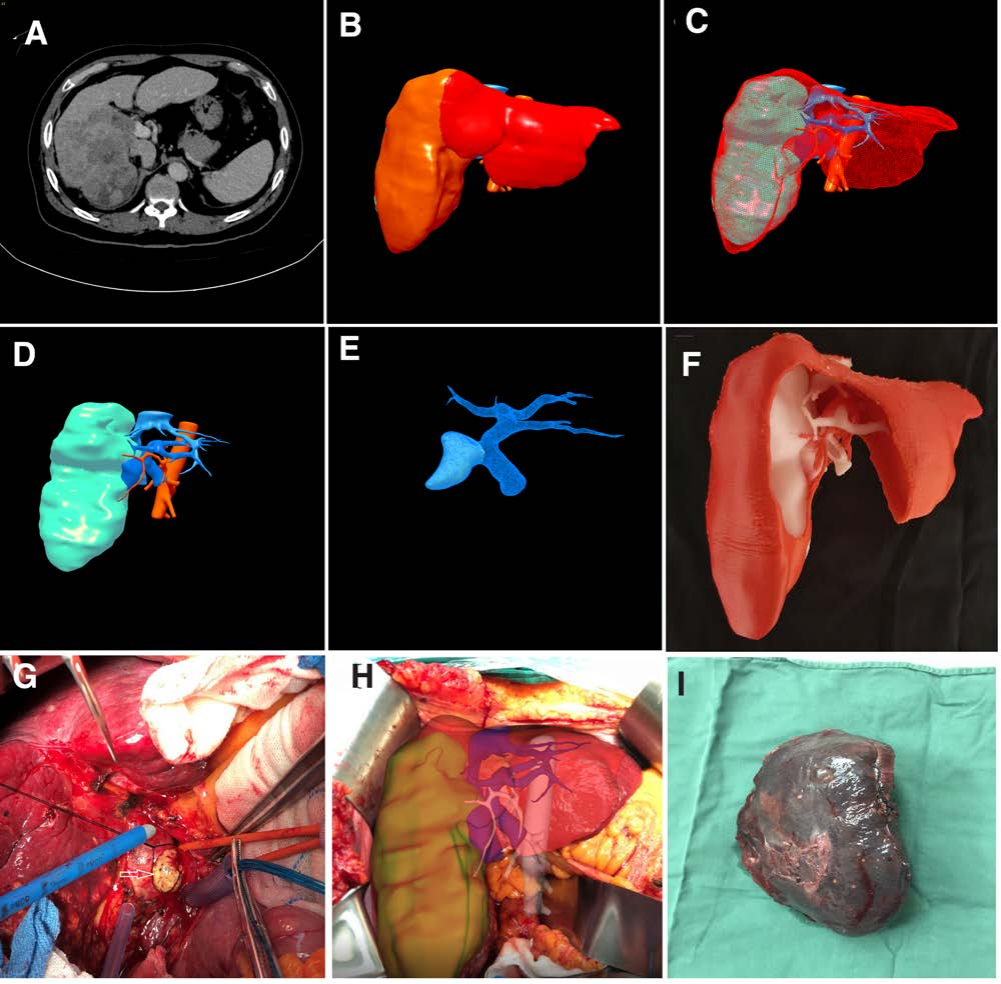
Preoperative evaluation and surgery navigation of liver cancer with portal vein tumor thrombus (PVTT). (A) The CT image showed right liver cancer with PVTT and liver fibrosis. (B) A three-dimensional (3D) reconstruction model showed the future liver remnant (FLR) was 508 mL and FLR/SLV (future liver remnant/standard liver volume) was 40.9% if right hemihepatectomy was performed. (C) Intrahepatic anatomy and tumor location Stereoscopic displayed by 3D visualization. (D) The relationship between tumor and hepatic artery, portal vein, and hepatic vein. (E) The classification of PVTT by 3D visualization (Cheng type Ⅱa or Japanese Vp classification Vp3). (F) Realistic liver anatomy with 3D printing model. The white part of the liver is composed of intrahepatic tumors, portal vein, and hepatic vein, while the red duct in the liver is the hepatic artery. (G) Portal vein tumor thrombectomy. (H) Fusion of 3D visualization image and intraoperative live scene. (I) The right liver tumor was completely removed.
